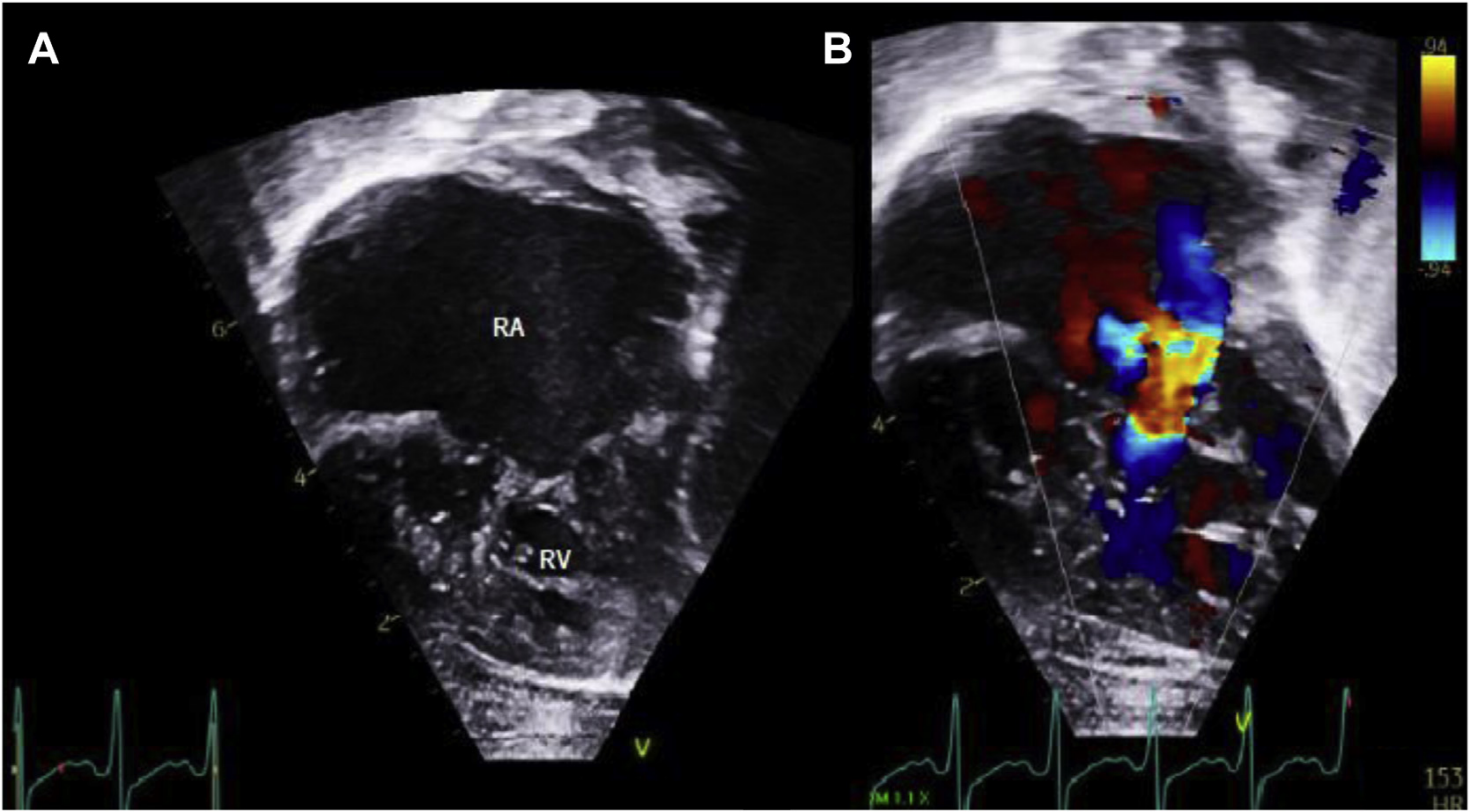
Echocardiogram demonstrating severe right atrial enlargement. A: Two-dimensional apical 4-chamber view demonstrating anatomy consistent with hypoplastic left heart syndrome. There is severe dilation of the right atrium. B: Apical 4-chamber view with color Doppler and severe tricuspid valve insufficiency owing to incomplete coaptation of valve leaflets. The severe tricuspid regurgitation led to severe right atrial dilatation, believed to be the nidus for incessant ectopic atrial tachycardia as well as heart failure. RA = right atrium; RV= right ventricle.
